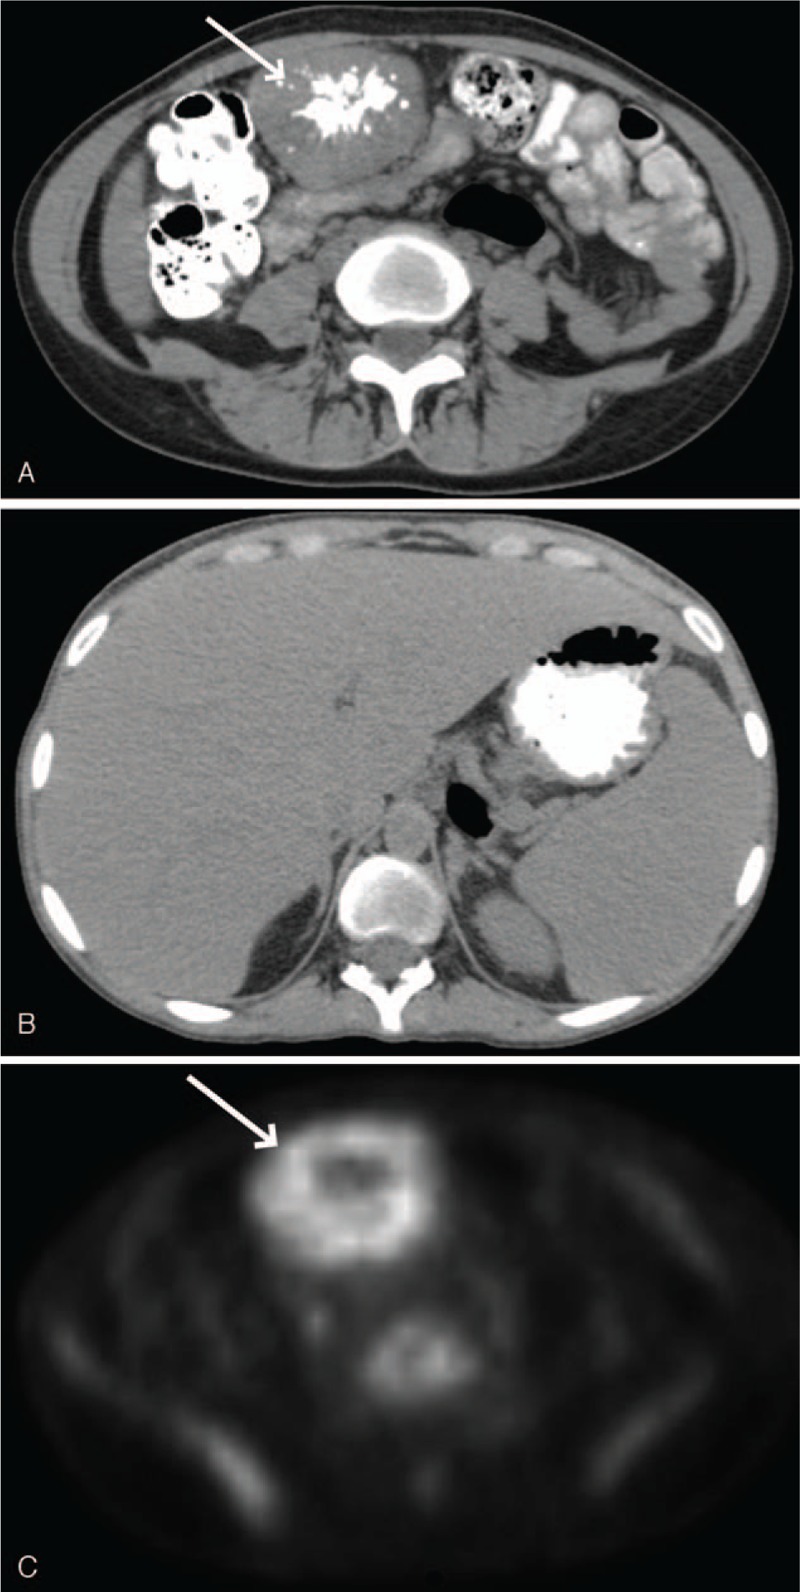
CT scan of the abdomen showing mesenteric mass (a; top), non-FDG avid hepatosplenomegaly (b; middle) and PET showing FDG avidity of the mass (c; bottom).
